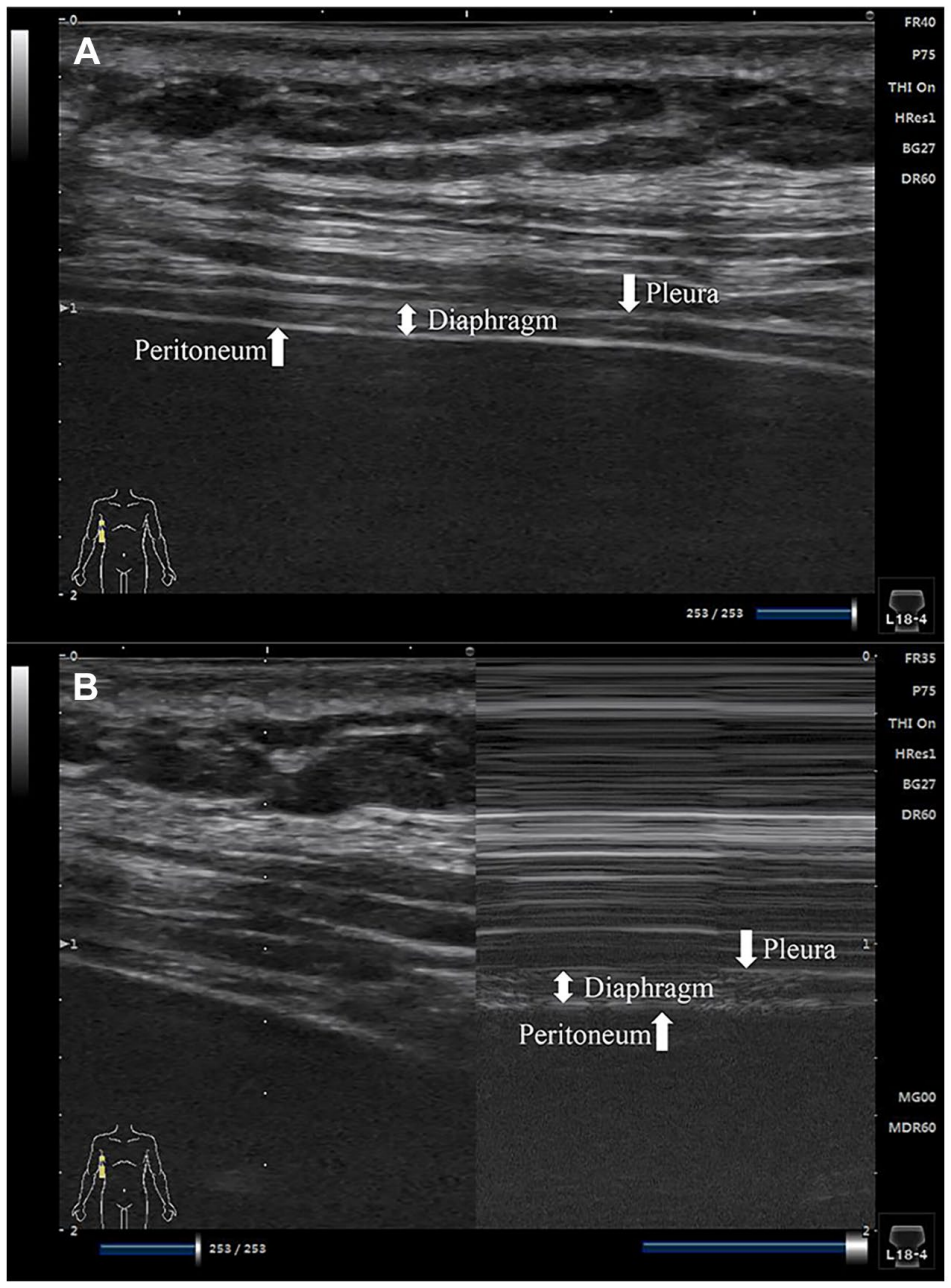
Images of the diaphragm ultrasound. (A) 2D mode diaphragm image; (B) M-mode diaphragm image.
